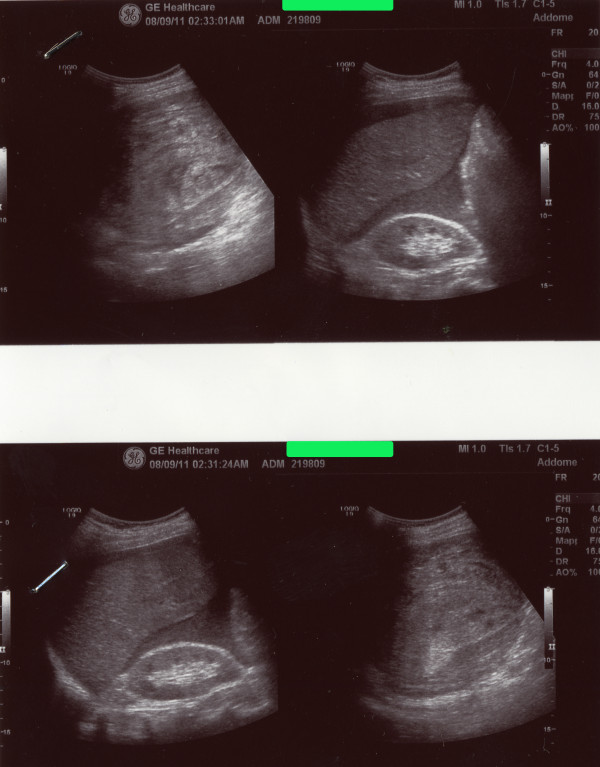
Abdominal ultrasonography showing the spleen rupture with hemoperitoneum.
